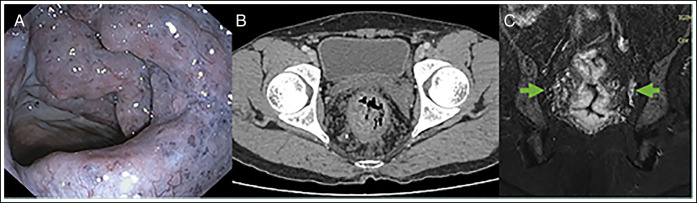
(A) Colonoscopy image showing bluish red elevated lesions occupying the complete circumference of the rectum. (B) Computed tomography scan showing diffuse thickening of the anorectal wall and phleboliths. (C) T2-weighted magnetic resonance imaging showing diffuse anorectal wall thickening and increased mesorectal vascularity (arrows).
